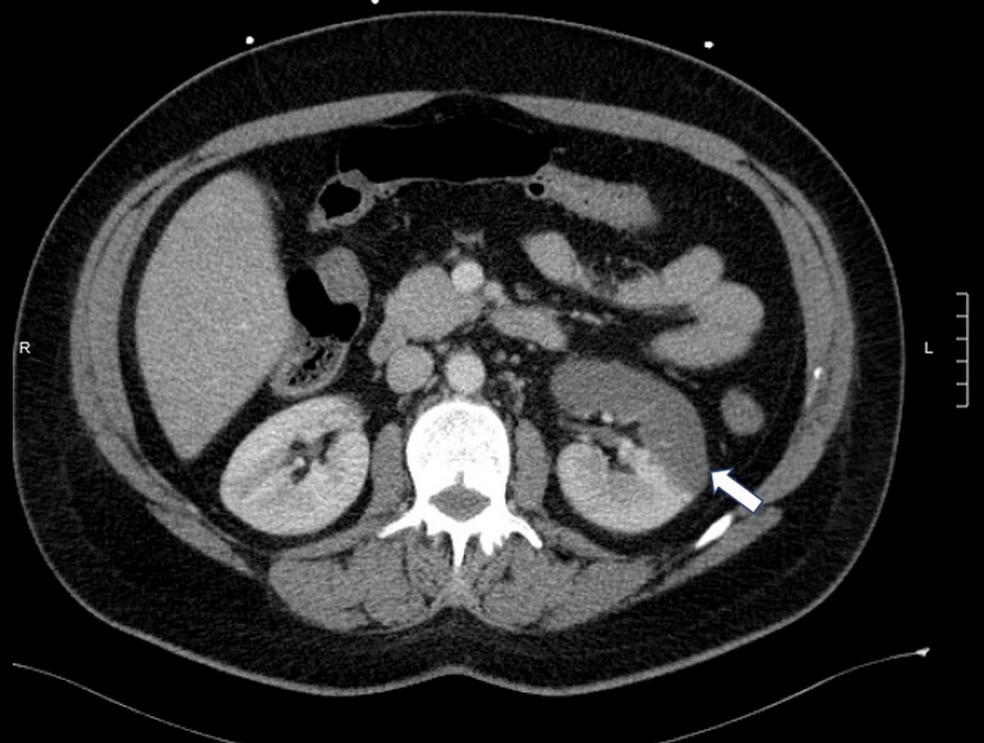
Axial computed tomography scan of the abdomen with contrast showing reduced enhancement of the anterior and caudal portion of the left kidney (white arrow) consistent with infarction.
